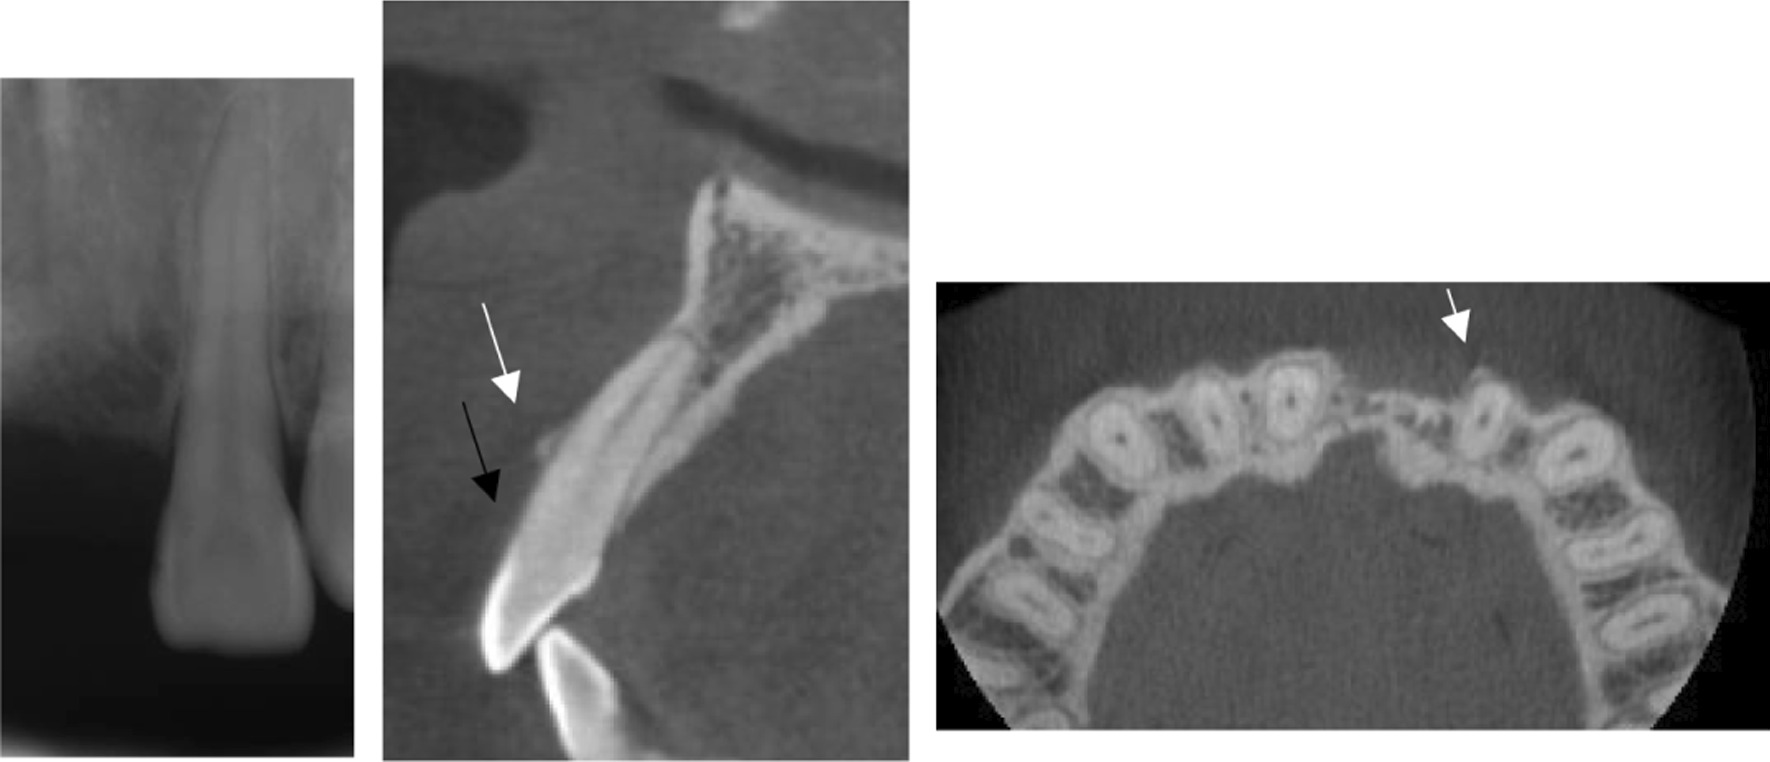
Periapical, sagittal and axial CBCT imaging. a No obvious interproximal alveolar bone resorption. b Area of bony dehiscence (black arrow) and bony exostosis (white arrow). c A bony exostosis in the cross section of cone beam CT (white arrow)
